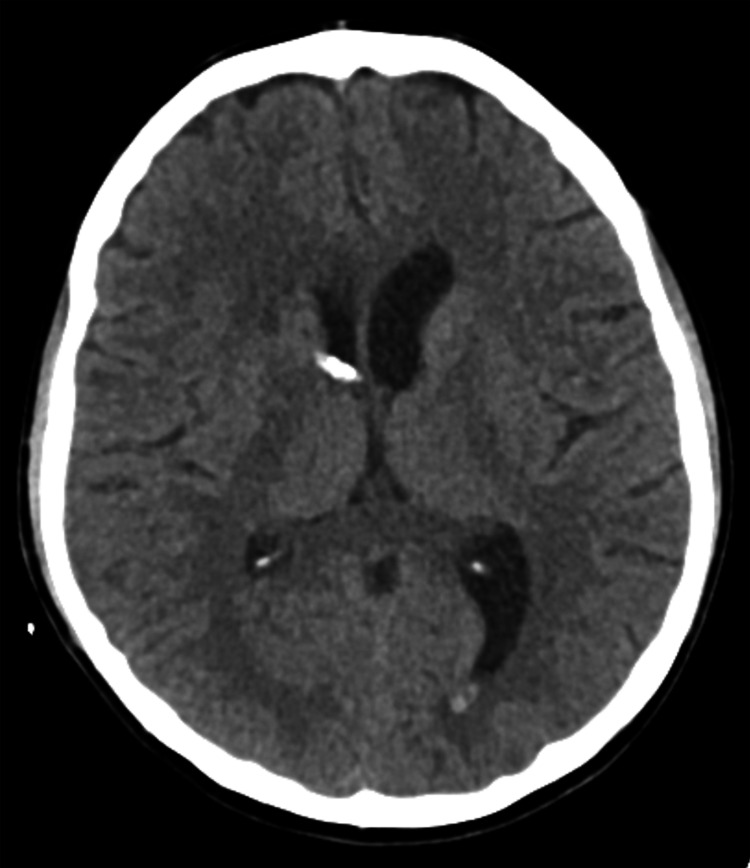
Imaging from postoperative day 11. The axial noncontrasted CTH demonstrates resolution of the intraventricular hemorrhage without evidence of infarction. CTH: computed tomography of the head
